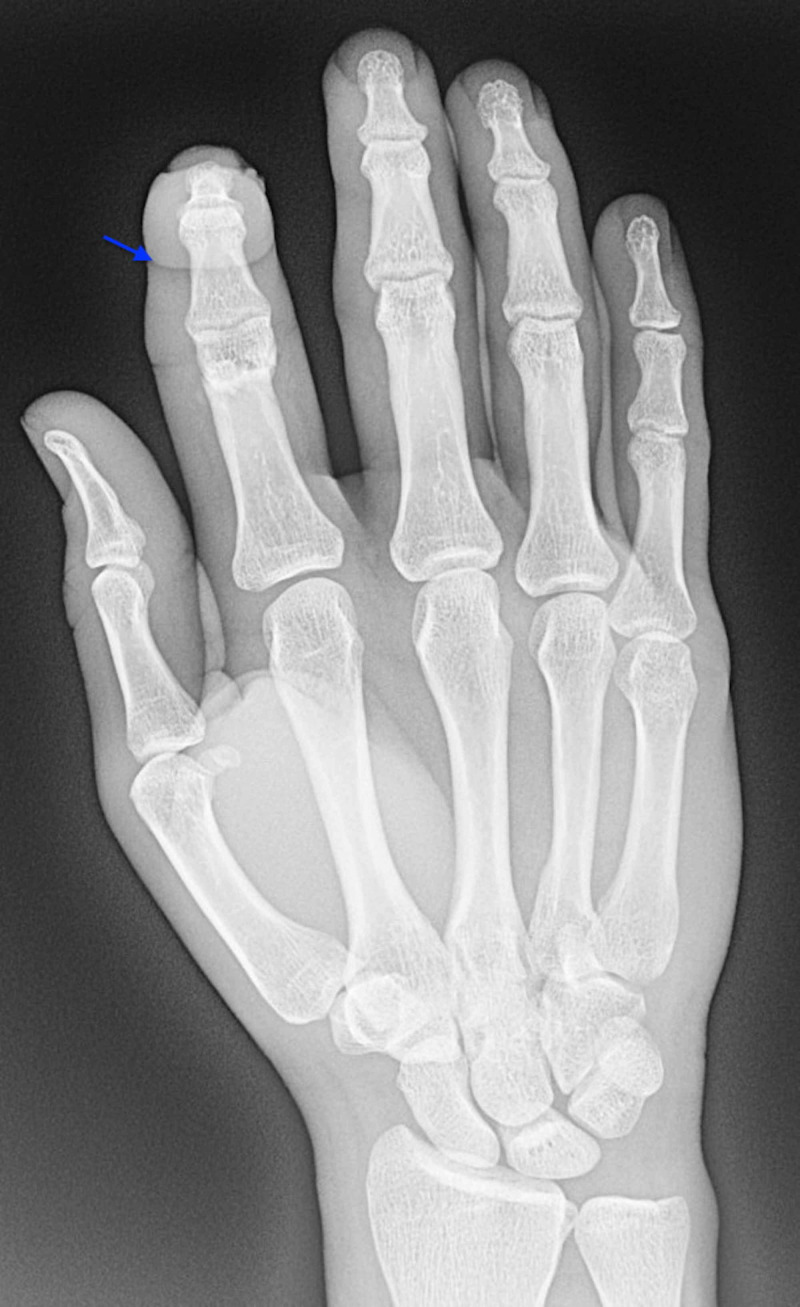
Posteroanterior plain radiograph of the right hand demonstrating flexion of the right index finger at the proximal interphalangeal joint
